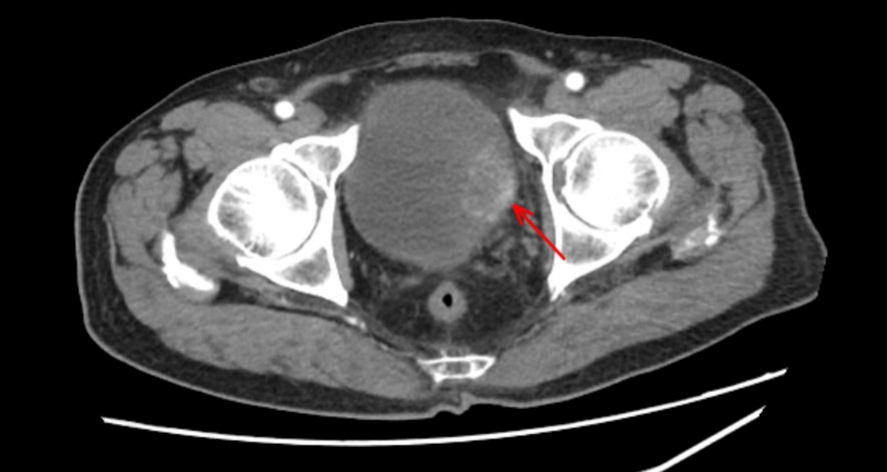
Preoperative CT scan demonstrating a space-occupying lesion within the bladder.
